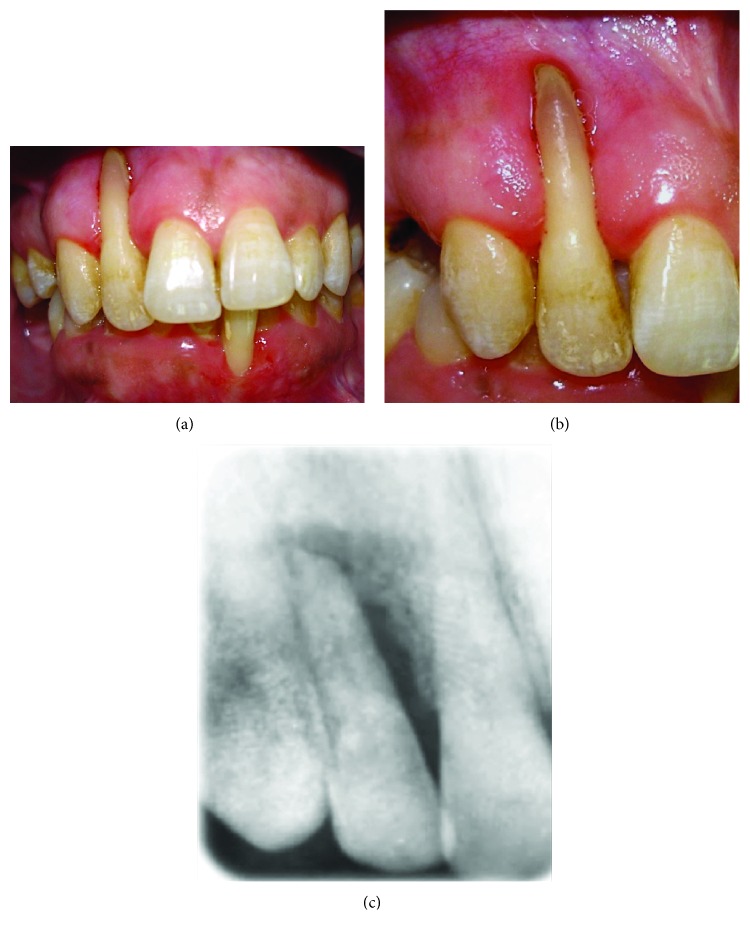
(a to c) Pretreatment clinical and radiographic images showing a deep Miller Class III GR of the tooth #2. Note a severe bone loss of the tooth and apex exposure.
